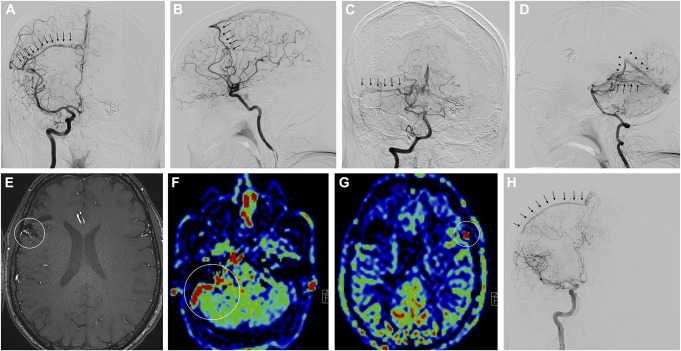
This patient underwent GKRS of a right frontal partially embolized AVM. A and B, Frontal and lateral internal carotid angiograms (arrows = early draining vein). C and D, Frontal and lateral angiograms of a ruptured AVM of the tectum after vertebral artery injection (arrows = early draining vein). E, MRI 121 weeks after GKRS showed persistence of the right frontal AVM nidus (circle) in time of flight, F and G, but in ASL only the right tectal AVM and the left frontal arteriovenous fistula were seen (circles). H, DSA confirmed early filling of the right frontal draining vein (arrows) after right internal carotid injection which was not seen on ASL. This was the only case of false-negative ASL. However, the overall result of the MRI protocol was true positive. ASL, arterial spin labeling; AVM, arteriovenous malformation; GKRS, Gamma Knife radiosurgery.
